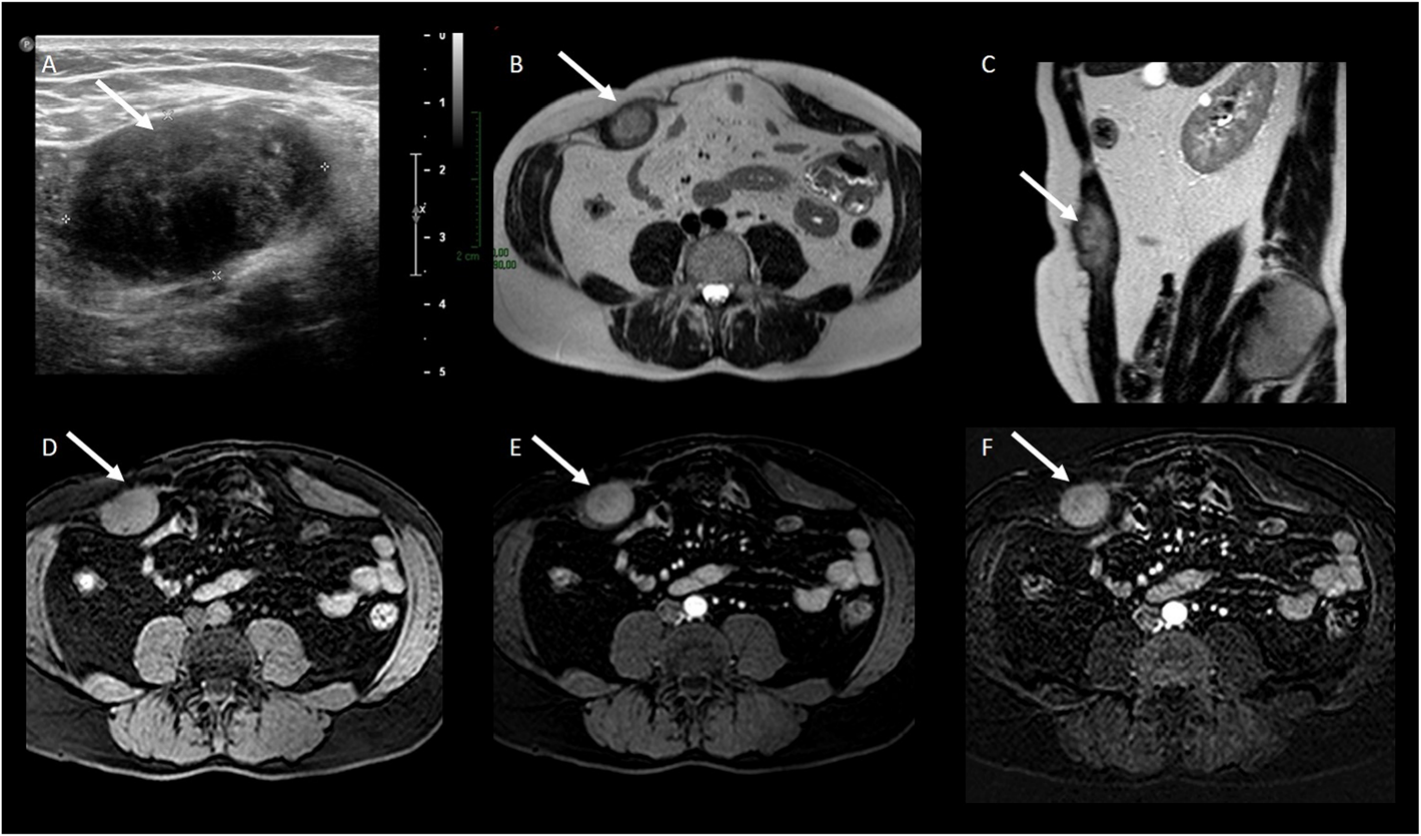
DT of the abdominal wall. a US examination showed a well-defined mass in the right rectus muscle. At MRI, the lesion is characterized by hyperintense signal on T2wi (b, c), isointense signal with the muscle on T1wi (d), and homogeneous enhancement on T1wi after contrast agent administration (e) especially on subtracted imaging (f)
